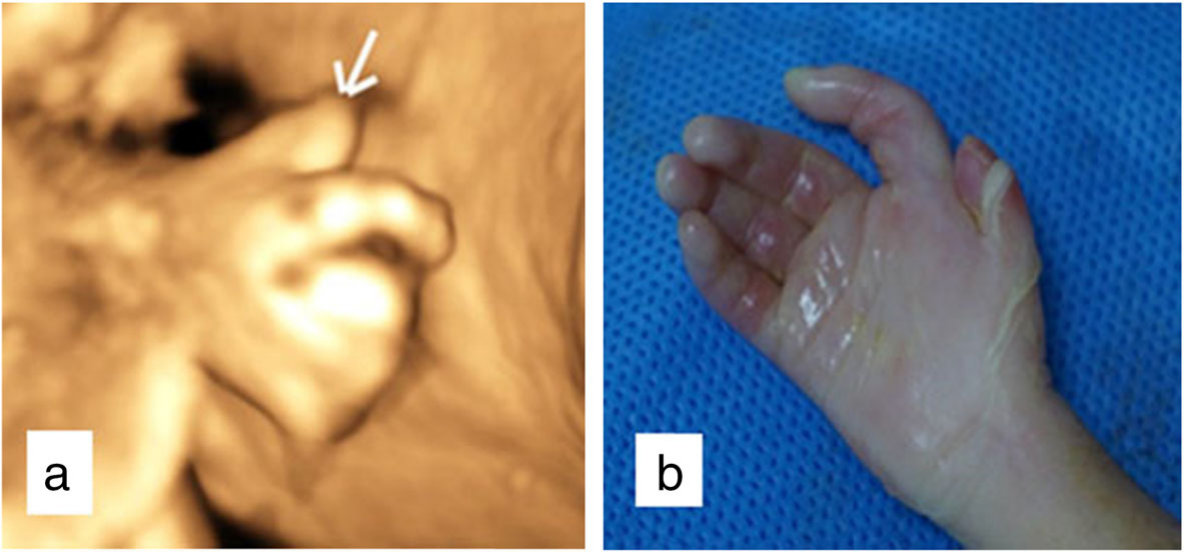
Brachydactyly of the right thumb. a prenatal 3D ultrasound b postpartum autopsy
